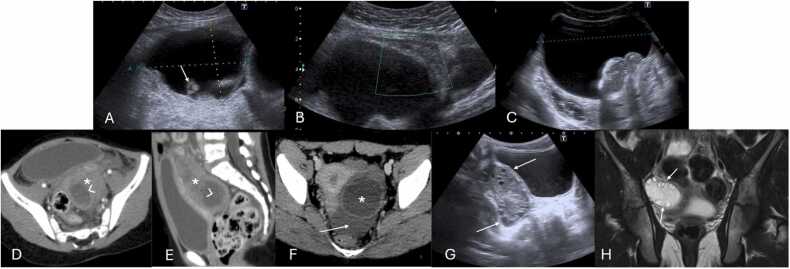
(A and B) 13-year-old female with left pelvic pain, surgically confirmed to be an ovarian torsion. Pelvic ultrasound revealed a large complex cyst (between calipers in A), with multiple thick septations (arrow), and no flow on Color Doppler (B). (C) Large complex mass (between calipers), predominantly cystic, with an internal solid component, in the left ovary, in a 14-year-old female, causing ovarian torsion. This mass was surgically confirmed to be a teratoma. (D and E) Contrast-enhanced pelvic CT of the pelvis of a 12-year-old female depicting an abnormally positioned, enlarged left ovary, containing a large cyst (arrowhead in D and E). Please also note, the loss of the normal enhancement pattern, indicative of torsion (* in D and E). (F) Another contrast enhanced pelvic CT on the axial plane, of a 14-year-old-female showing an enlarged, abnormally positioned, left ovary (*) due to the presence of a large simple cyst (*) and associated free fluid in the pouch of Douglas (arrow). (G and H) Pelvic transabdominal ultrasound (G) and Coronal T2-weighted image of an 11-year-old female with surgically proven right ovarian torsion, depicting heterogeneous ovary appearance with associated peripheral displacement of follicles by stromal edema (arrows).
